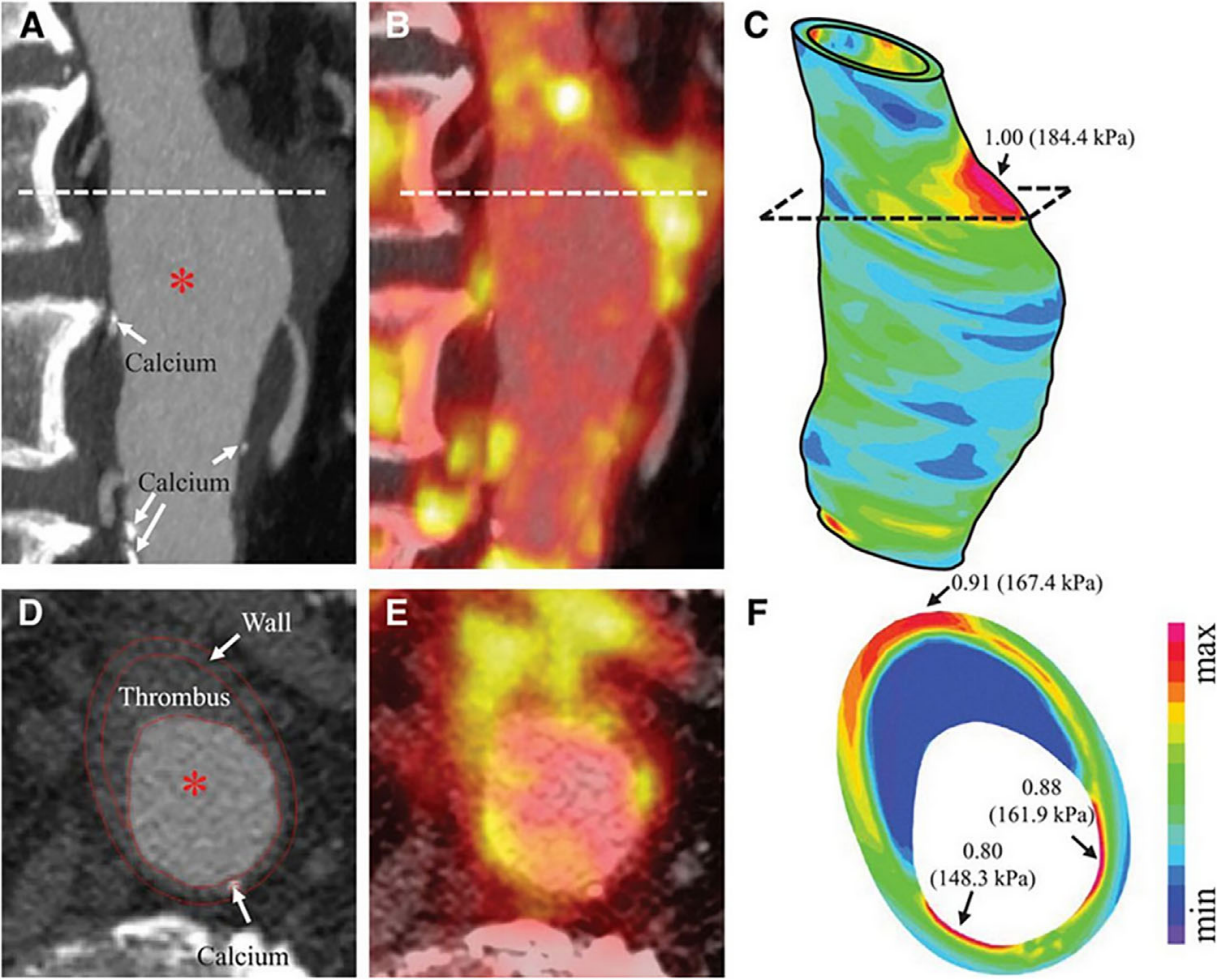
In vivo imaging and structural stress of abdominal aortic aneurysms (AAA). Sagittal‐view (A) contrast‐enhanced CT and (B) [^18^F]FDG PET, with (C) structural stress plotted on 3D geometry. Transverse‐view (D) contrast‐enhanced CT and (E) [^18^F]FDG PET, with (F) structural stress plotted on the transverse plane.^13^ Figure license: Huang et al., 2016. High structural stress andpresence of intraluminal thrombus predict abdominal aortic aneurysm 18F‐FDGuptake. This article is distributed under the terms of the Creative CommonsAttribution 4.0 International License (https://creativecommons.org/licenses/by/4.0/). Abbreviations: AAA, abdominal aortic aneurysm; CT, computed tomography; [^18^F]FDG, [^18^F]fluorodeoxyglucose; PET, positron emission tomography.
